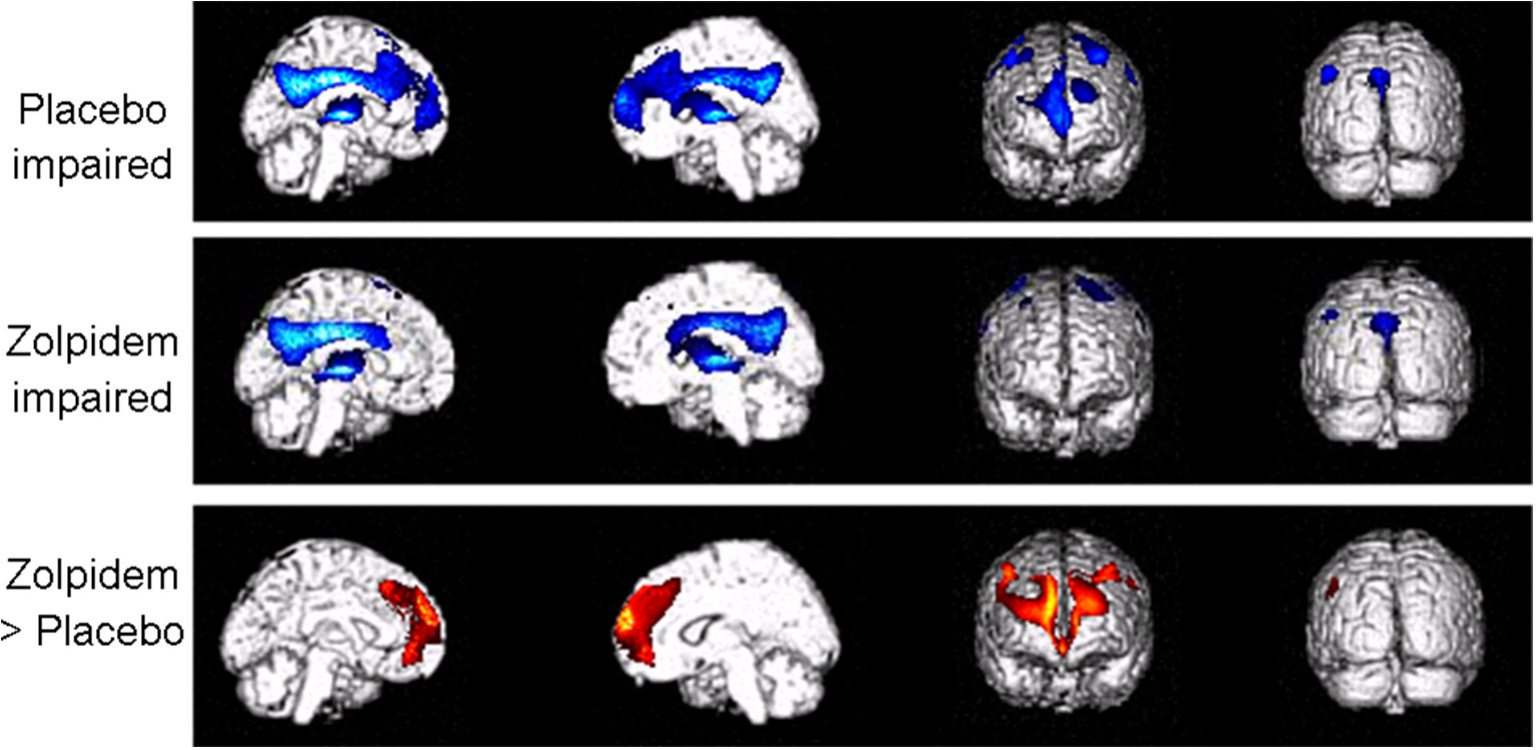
Impaired brain metabolism after placebo and zolpidem intake and areas showing relative recovery after zolpidem. Brain areas showing impaired metabolism (in blue) following placebo and zolpidem administration and regions which were impaired following placebo but showed relative recovery of activity after zolpidem intake (in red). For display purposes results are shown thresholded at uncorrected *p* < 0.001. From left to right, medial right and left view, frontal and posterior view of a 3D rendered brain MRI.
